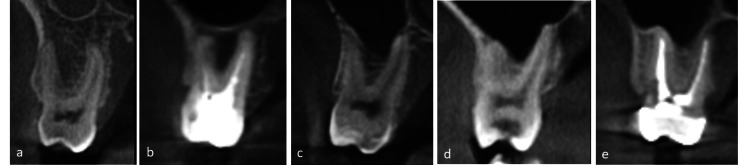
Cone-beam computed tomography Coronal sections showing proximity of the root tips of posterior maxillary teeth (RPMT) to the sinus floor: a) Type 0: no contact with the sinus cortical borders; b) Type 1: the root tips are in contact with the cortical borders of the sinus with an inferiorly curving sinus floor; c) Type 2: the root tips are projecting laterally on the sinus cavity, but the apex is outside the sinus boundaries with an inferiorly curving sinus floor; d) Type 3: the root tips are projecting into the sinus cavity with an inferiorly curving sinus floor; e) Type 4: a superiorly curving sinus floor enveloping part or all of the root tips.
